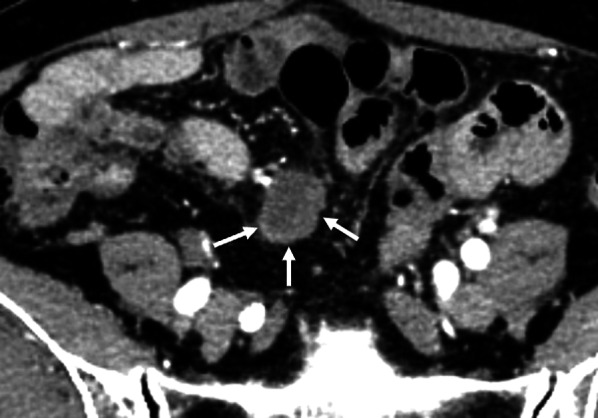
Enhanced computed tomography image showing a 23-mm low-density tumor in the abdominal cavity (white arrows)
